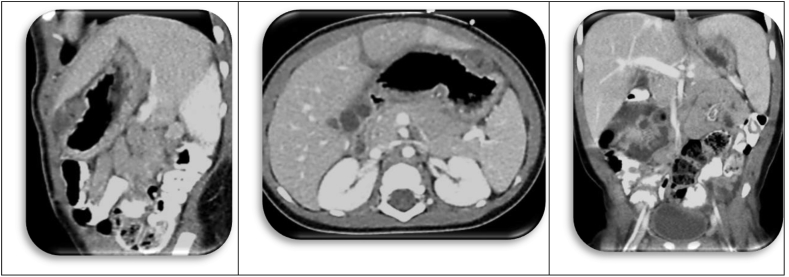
CT scan Showing Recurrence in Stomach and Liver.
